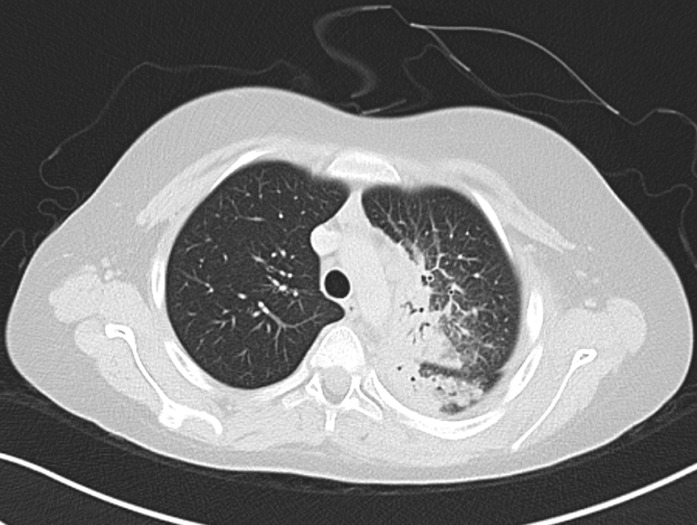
CT chest showed multiple left hilar lymph nodes with collapse of the left upper lobe and multiple pulmonary nodules with cavitation
